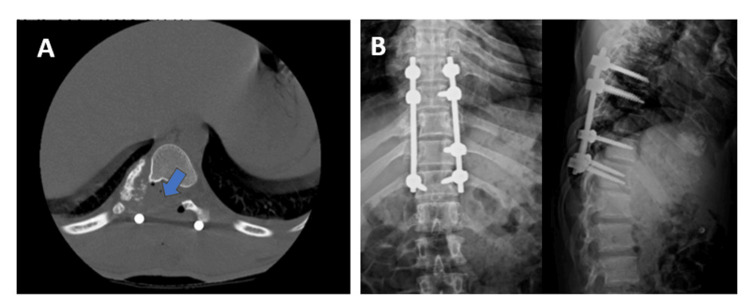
Postoperative CT and radiographs. (A) CT scan: Blue arrow showing the absence of lesion in the spinal canal. (B) AP and lateral spine radiographs: To confirm the position of the pedicular screws. AP: anteroposterior; CT: computed tomography.
